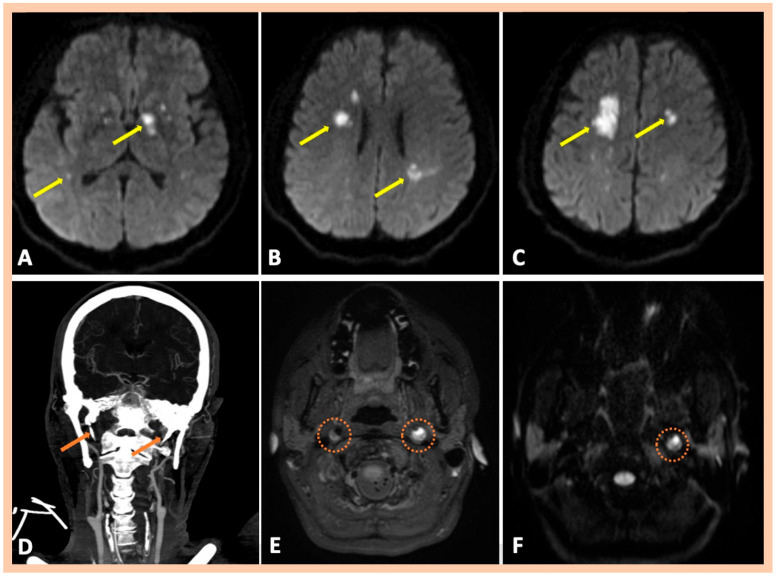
Neuroimaging findings—Patient #10. DWI sequence revealing multiple acute ischemic lesions with diffusion restriction in bilateral anterior circulation territories (Panels (A–C)). Computed tomography of the neck and brain revealed elongated styloid processes of the stylohyoid ligaments bilaterally (Panel (D)). Three-dimensional fat-saturated black-blood T1-weighted high-resolution (3 Tesla) vessel wall imaging showing crescent-shaped, hyperintense lesions in the vessel wall of the right ICA in the subacute phase (Panel (E)) and of the left ICA in the acute phase (Panel (E)). A characteristic crescent- or ring-shaped lesion with DWI restriction is detected along the affected segment of the left ICA (Panel (F)).
